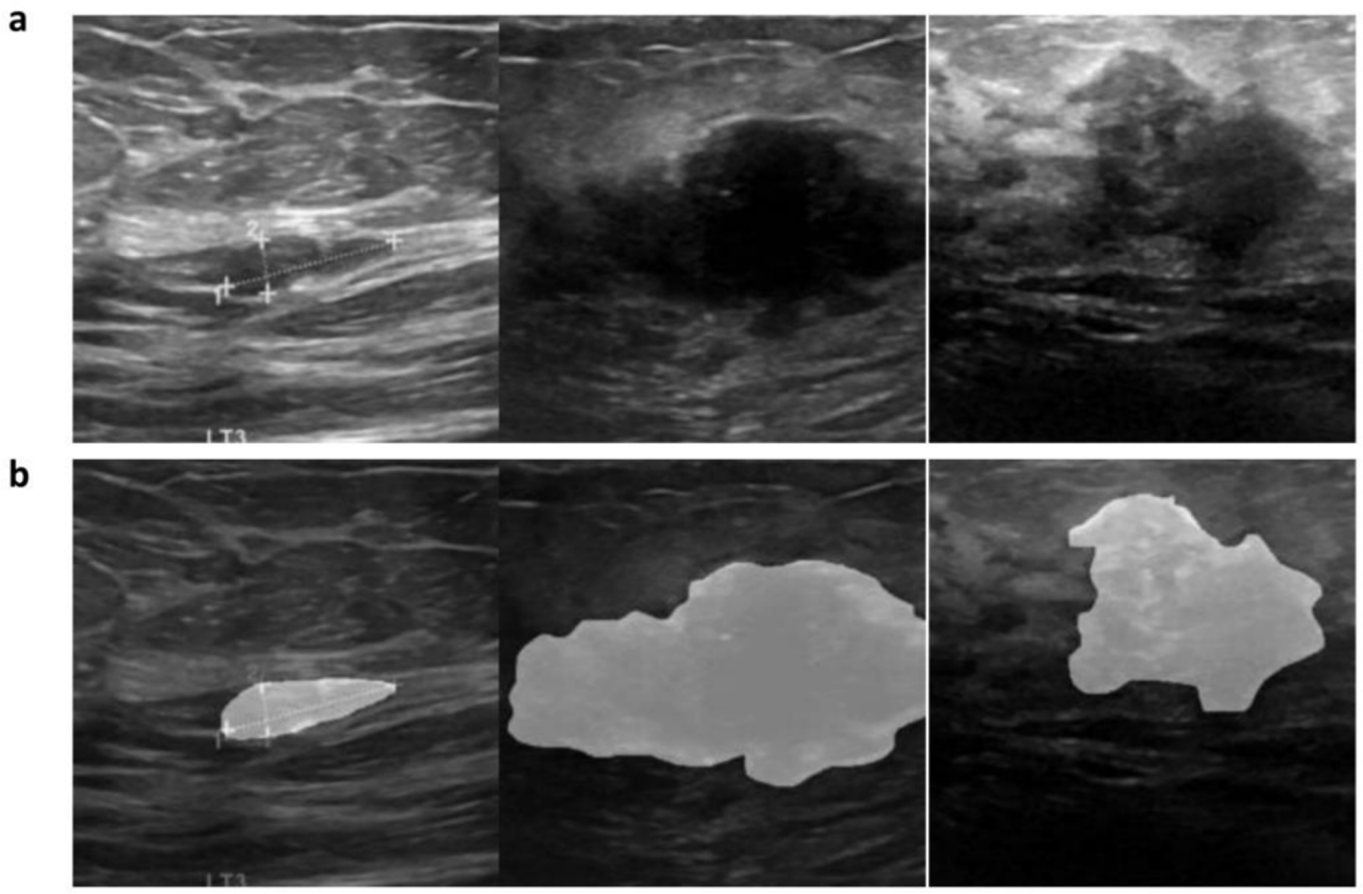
Data Annotation: Radiologist-Segmented Lesion ROIs. a. The first column shows the original ultrasound images. b. The second column displays the images with integrated lesion ROI (Region of Interest) results.
